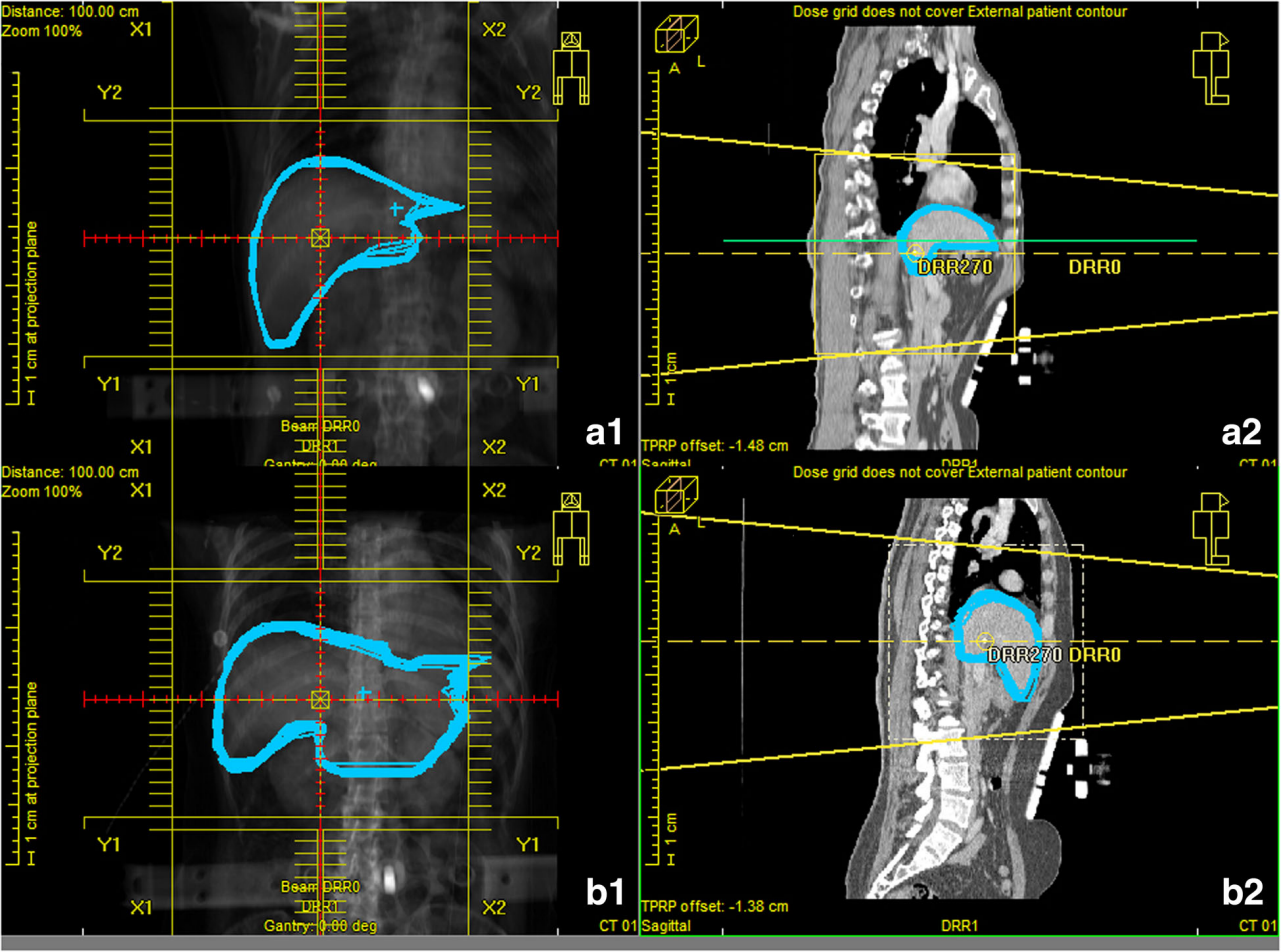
Overlay of 10 liver contours rendered on a digitally reconstructed radiographic image showing the relationship between body mass index and breath amplitude in the 3-dimensional directions from a qualitative perspective. The image in a1 is a tight overlay of 10 liver contours for a patient with a normal body weight (a2), and the image in b1 is a loose overlay for an overweight patient (b2)
